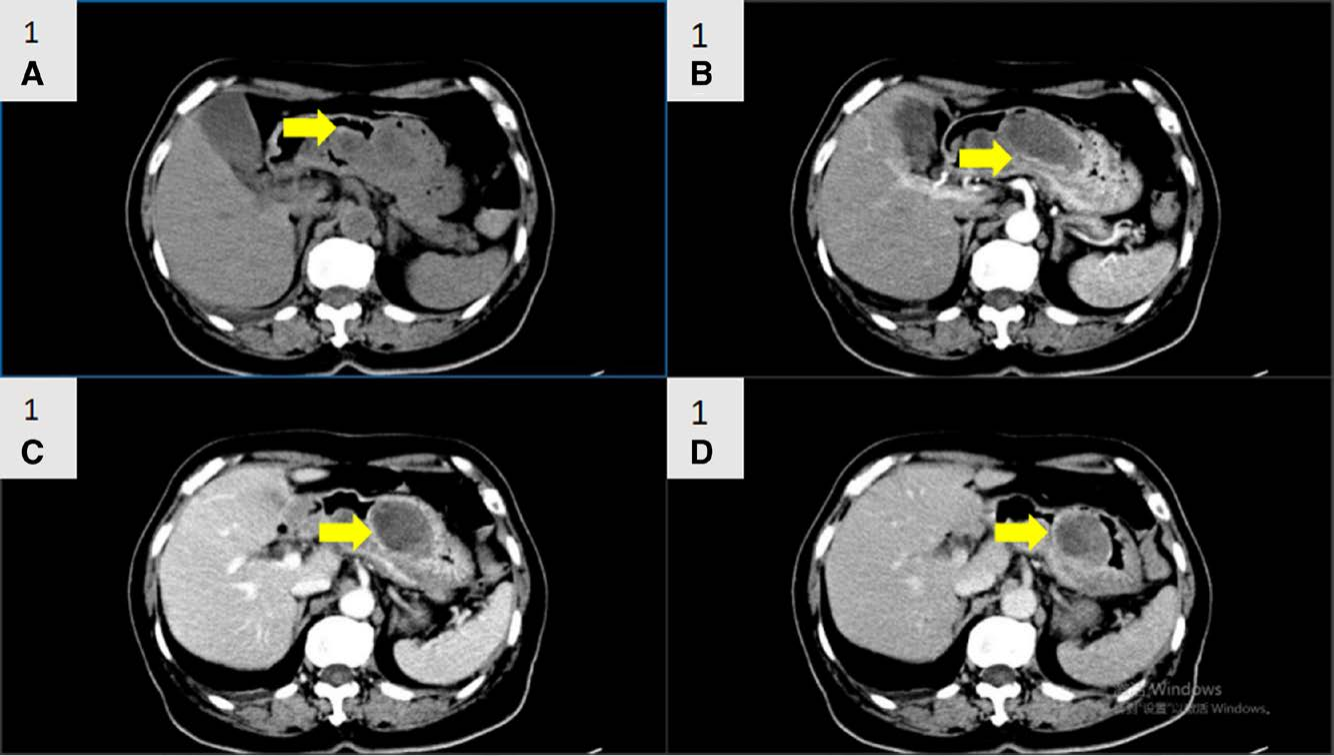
Contrast-enhanced computed tomography. (A–D) The CT revealed a nodule (yellow arrow) with high-density shadow in the gastric body, and ring enhancement in enhanced scan with clear boundaries.
